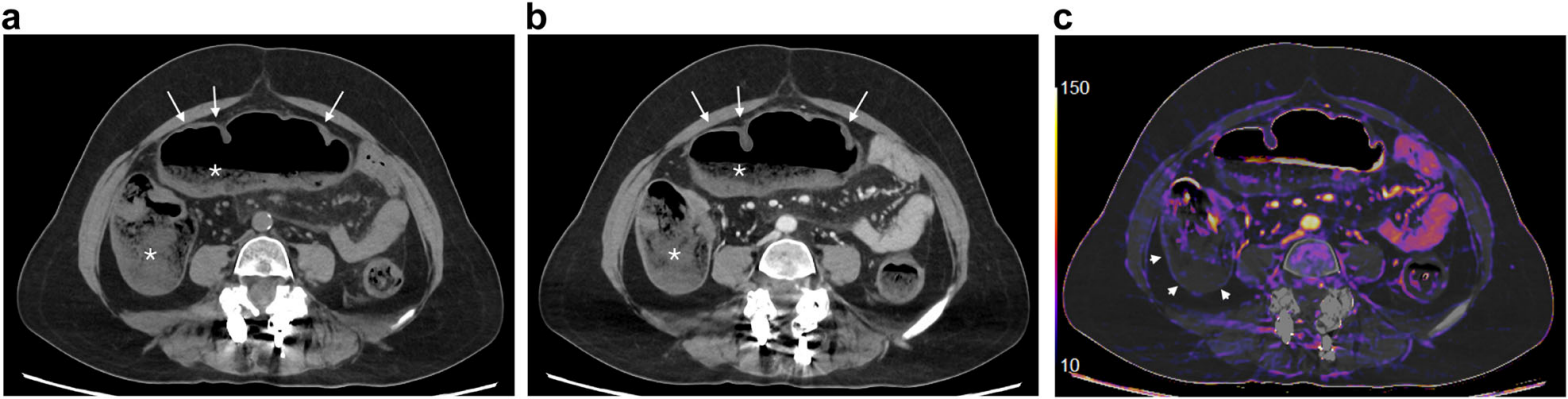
Ischemic colitis complicating fecal impaction. Axial unenhanced and portal phase CT (a, b) in an 80-year-old woman shows compacted feces in the right and transverse colon (asterisk), associated with a smooth annular wall thickening (arrows), suggesting ischemic colitis due to the distension. Axial subtraction sequence (c) confirms a lack of enhancement of the right colonic wall (arrowheads)Damages on the bowel lumen with LBO. CT is useful for identifying the mottled pattern due to feces and for assessing the proximal site of the impaction in order to facilitate management through disimpaction techniques, i.e., digital manipulation, disimpaction under anesthesia, or water-soluble contrast enema for more proximal FI [52]. The continuous contact between feces and wall may cause mucosal irritation, resulting in an increase in mucous secretion and paradoxical diarrhea [50].Damages on the adjacent structures mainly occur on the urinary system with bladder retention and obstructive uropathy [50].
